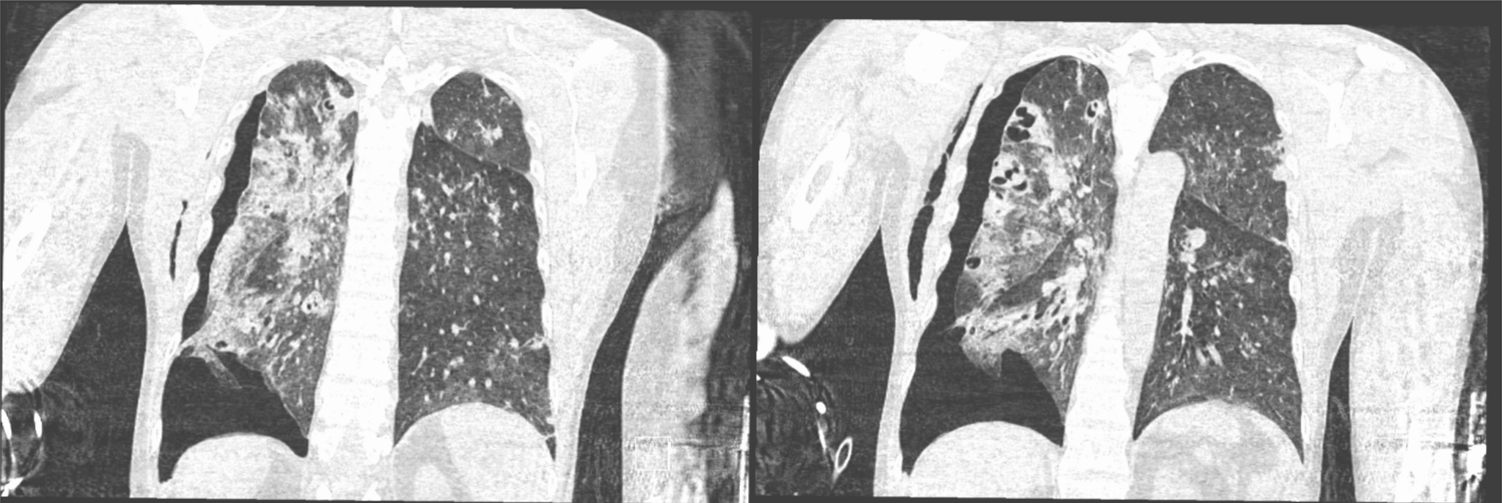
Lung computed tomography shows multiple pulmonary balloon lesions, and a *Staphylococcus aureus* infection was considered.
